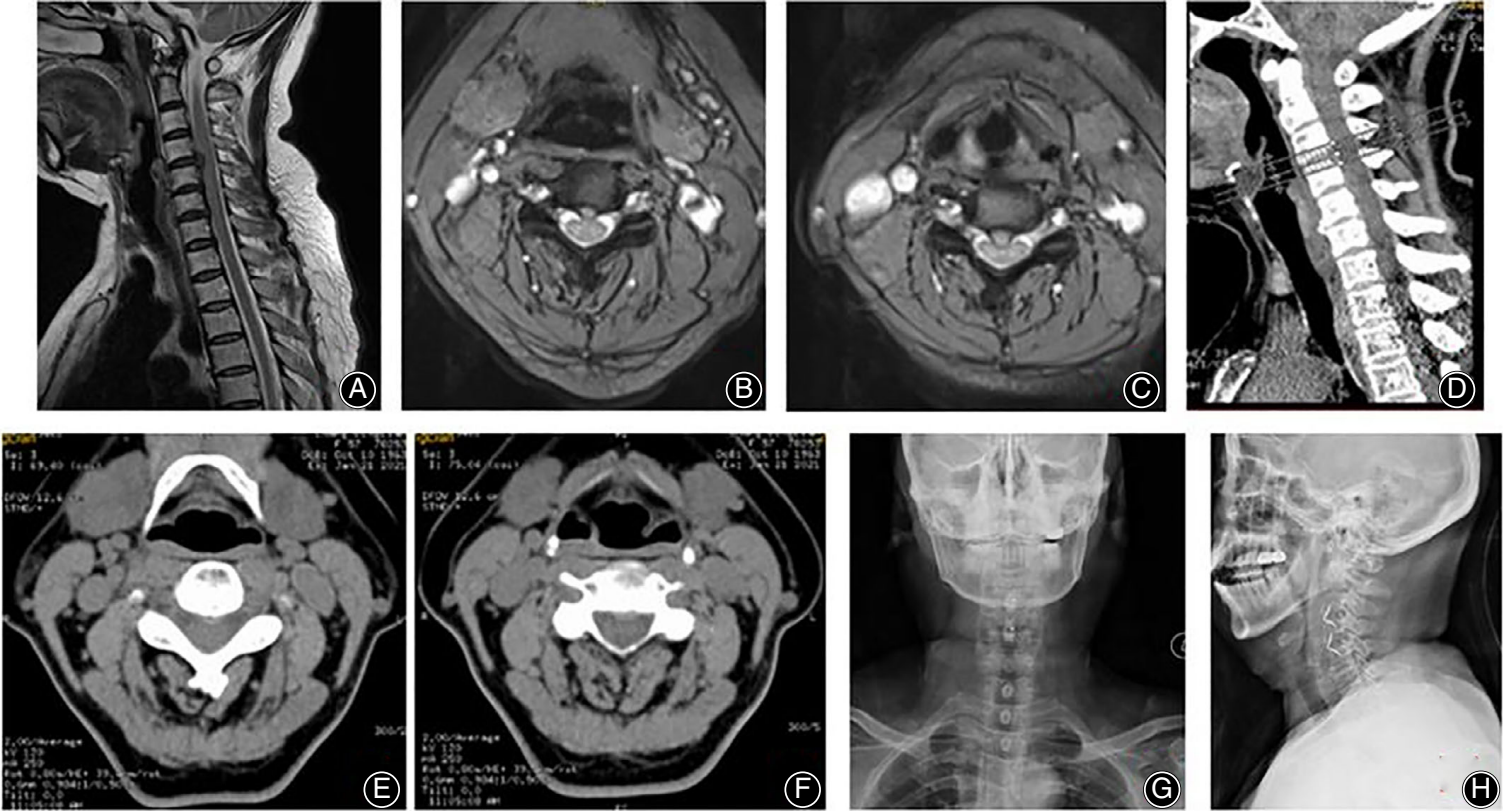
A 57‐years female patient with cervical spondylosis myelopathy. The patient had mild dysphagia after surgery, and the symptoms disappeared 3 months after surgery. (A–C) MRI showed herniated discs at C_3/4_ and C_4/5_ with spinal cord compression. (D–F) CT showed no calcification of OPLL or intervertebral disc. (G–H) X‐ray showed a satisfactory fixation position at 2 years of follow‐up.
